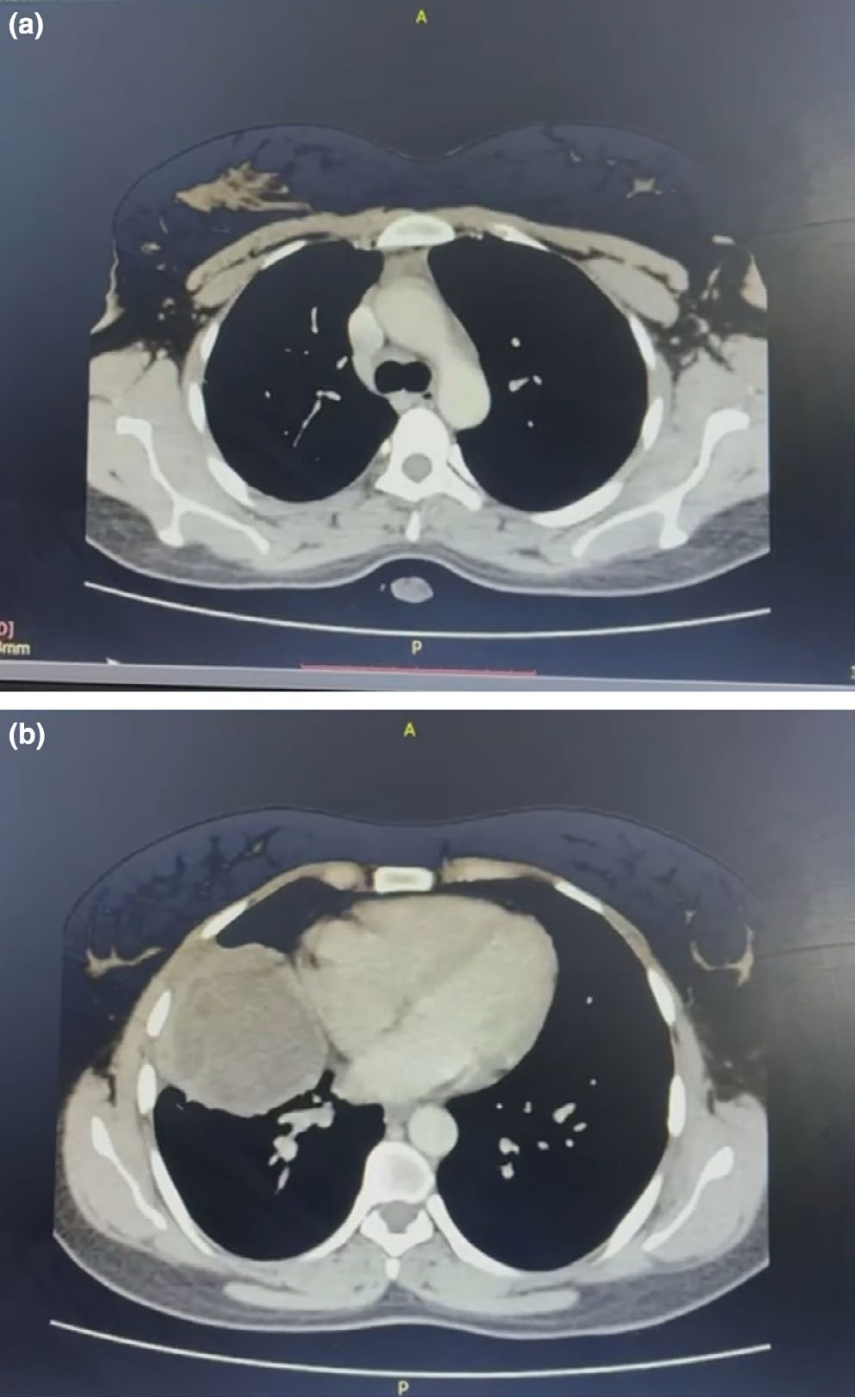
(a) CT scan of the thorax: Axial view showing a middle lobe lesion with peripheral enhancement and a central membrane, suggestive of a hydatid cyst. (b) CT scan of the thorax: Axial view showing a middle lobe lesion with peripheral enhancement and a central membrane, suggestive of a hydatid cyst.
